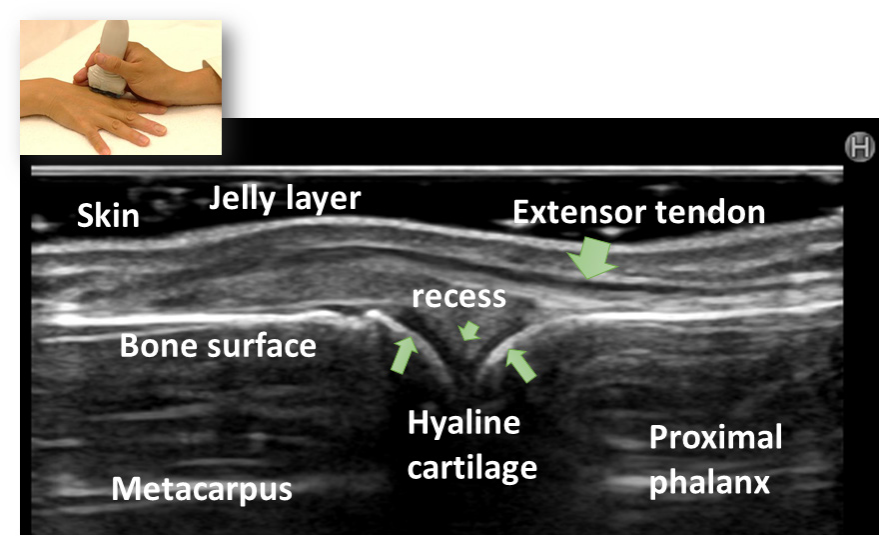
Ultrasonography can visualize comprehensive musculoskeletal tissue (healthy, dorsal metacarpal phalangeal joint). Ultrasound generates high-resolution images depending on the reflection rate of each musculoskeletal anatomical structure and almost completely reflects the bone surface. The joint cavity could not be identified in healthy subjects.
